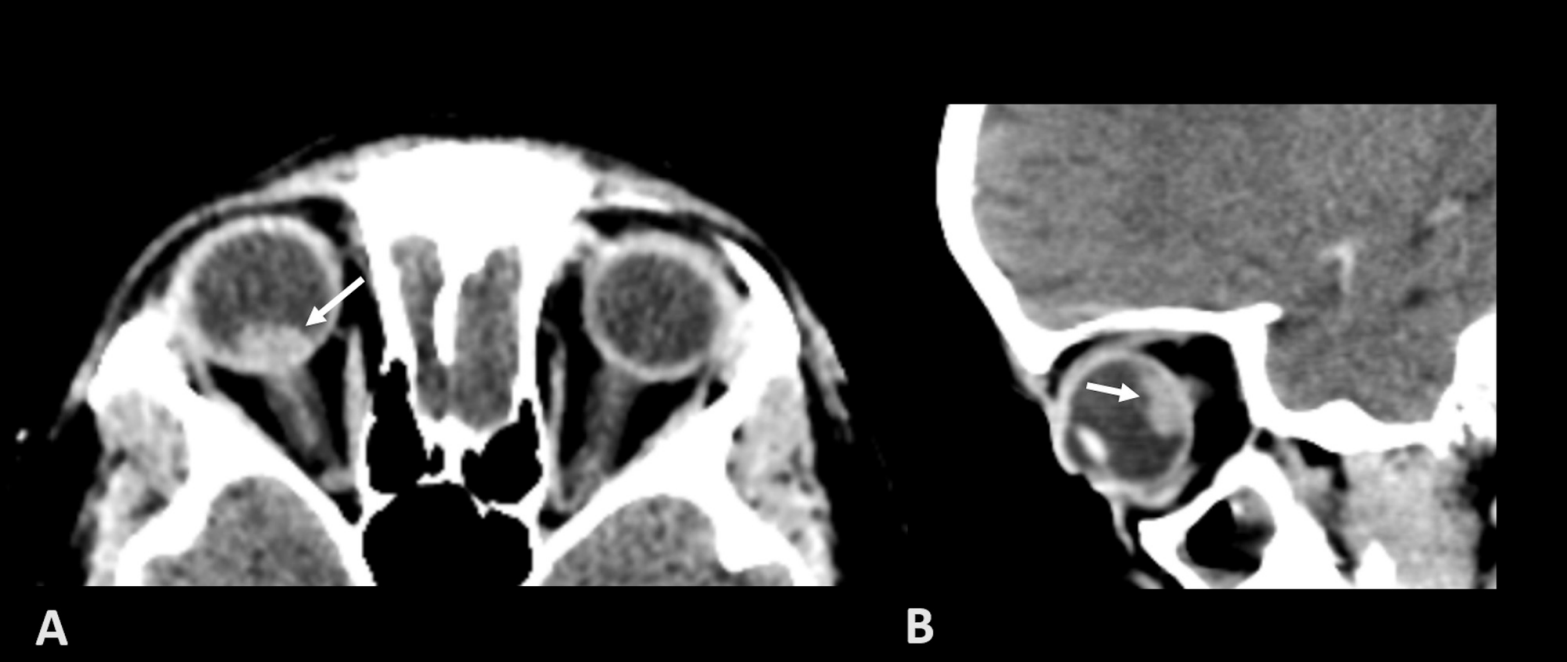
Axial (A) and Sagittal (B) post-contrast CT of the orbits shows a spontaneously hyperdense mass in the posterior aspect of the right globe (white arrows).  The left eye is unremarkable.
